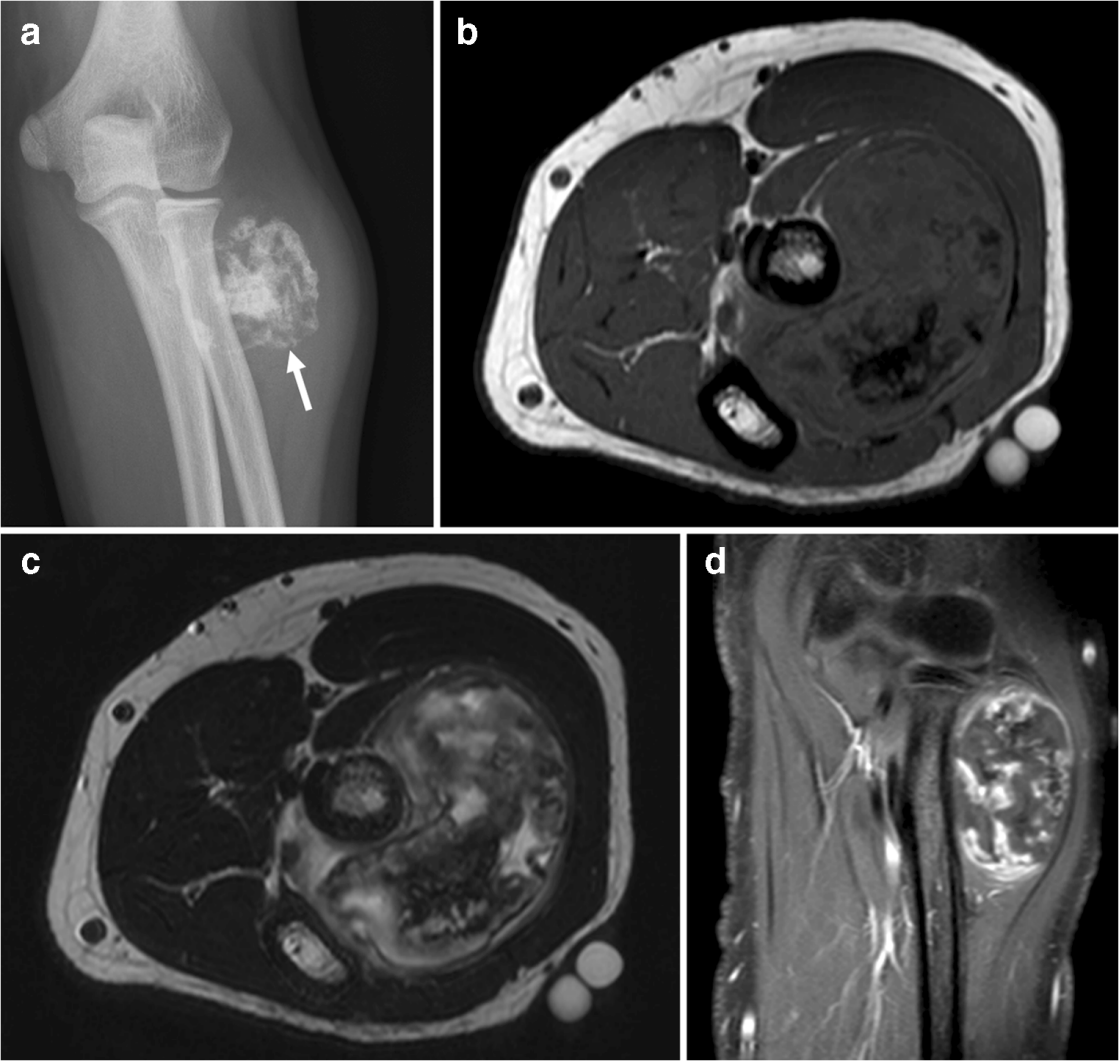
A 16-year-old boy with periosteal chondroma. a Radiography shows a calcified chondroid matrix laterally to the proximal radial diaphysis. b, c Axial T1-weighted (a) and T2-weighted (b) images show that the lesion is located directly adjacent to the radius. Signal intensity of the lesion is nearly isointense to muscle on T1-weighted images and hyperintense to T2-weighted images, with areas of signal loss which represent the calcified chondroid matrix. d Coronal postgadolinium T1-weighted image with fat suppression shows a septal enhancement pattern
